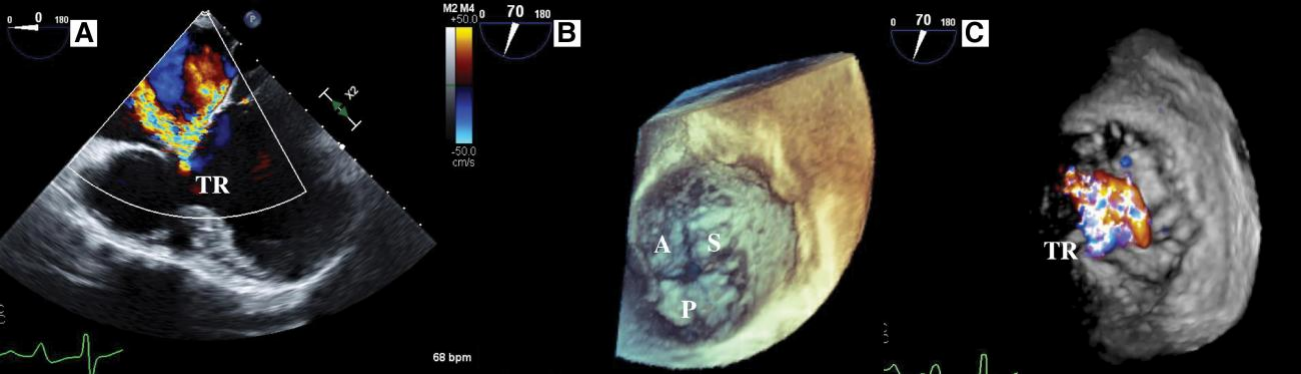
Baseline transesophageal echocardiography images. (*A*) 2D transesophageal echocardiography pre-procedural imaging demonstrates severe tricuspid regurgitation. (*B*) 3D transesophageal echocardiography imaging shows poor tricuspid valve coaptation between the anterior and septal tricuspid leaflets. (*C*) Severe tricuspid regurgitation originating from the anterior/septal tricuspid leaflets. TEE, transesophageal echocardiography; TR, tricuspid regurgitation; A, anterior tricuspid leaflet; S, septal tricuspid leaflet; P, posterior tricuspid leaflet.
